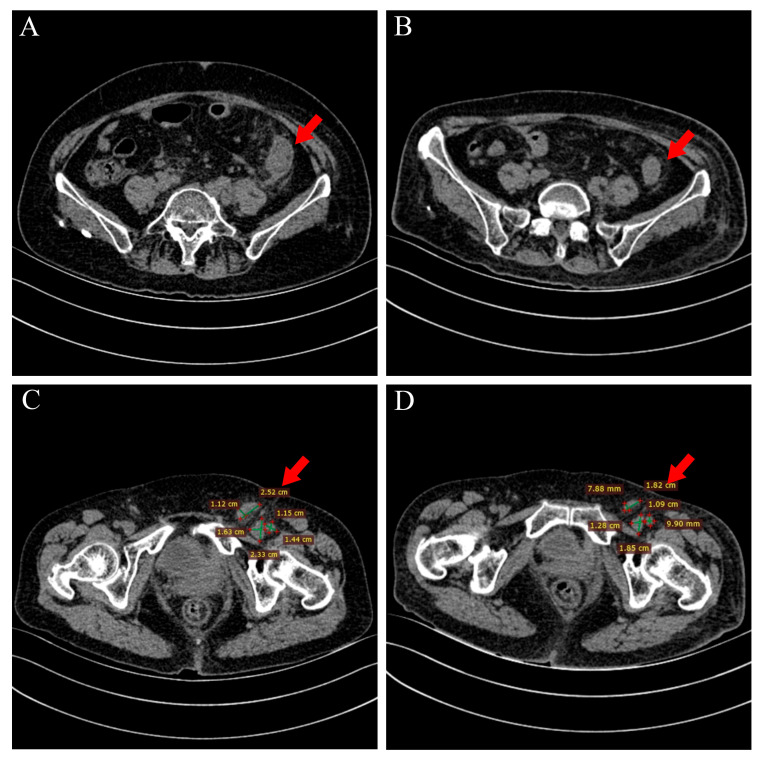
Whole-abdominal non-contrast CT scan. (A) Pre-treatment axial image shows circumferential wall thickening of the descending colon and increased pericolonic fat stranding. (B) Follow-up axial CT scan at the same level after two cycles of GC regimen shows marked regression of colonic wall thickening. (C) Baseline scan shows an enlarged left inguinal lymph node, largest dimension 2.52 × 1.63 cm. (D) Post-chemotherapy scan at the same level shows significant lymph node reduction, largest dimension 1.82 × 0.79 cm.
